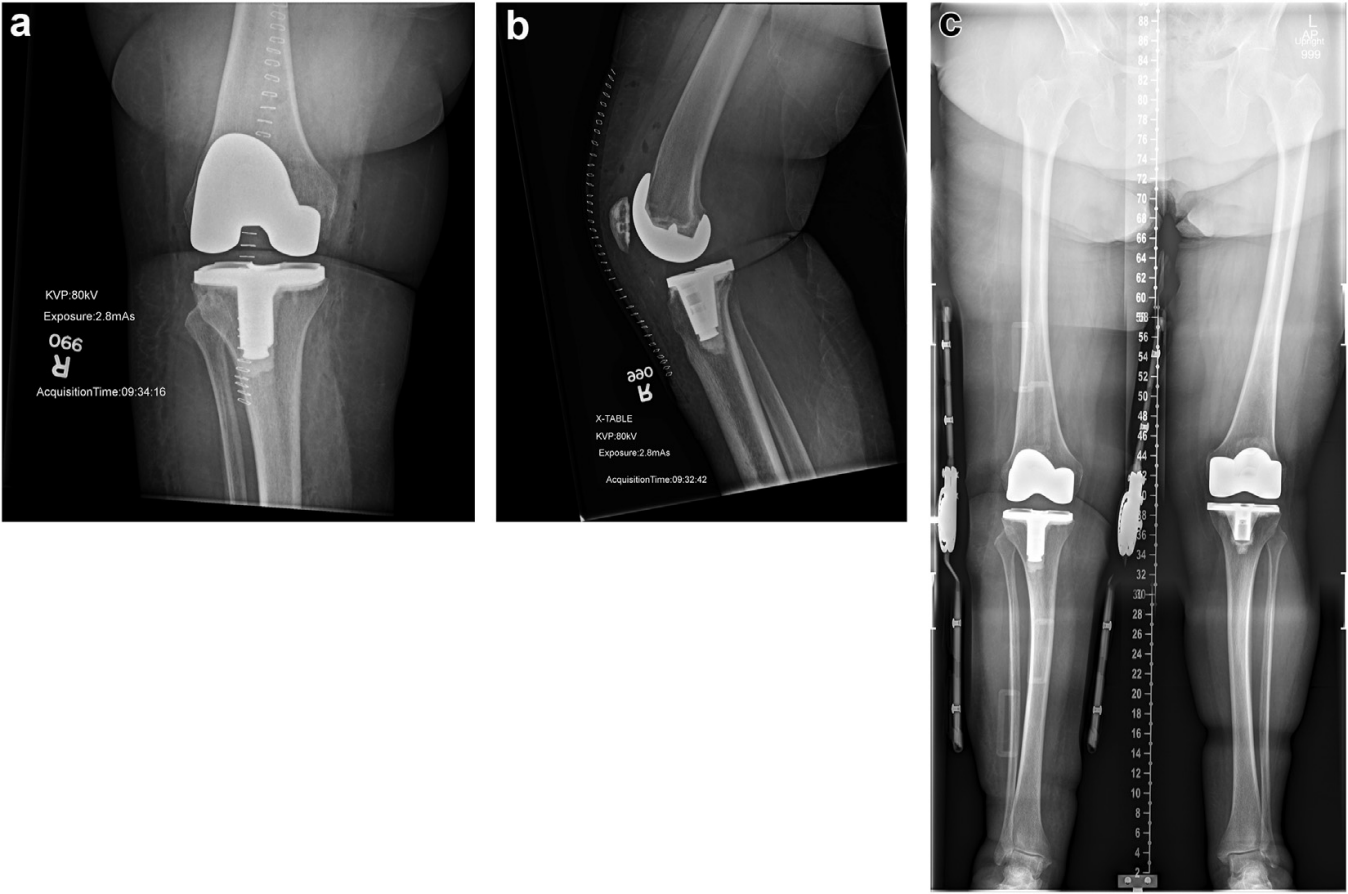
First right total knee arthroplasty (TKA) revision, immediately postoperatively. (a) AP and (b) lateral radiographic views demonstrate satisfactory alignment. Postoperative posterior slope measured at 6.2 degrees, clinically unchanged from preoperative measurement of 6.1 mm. Longstanding AP view of both legs at office follow-up (c).
